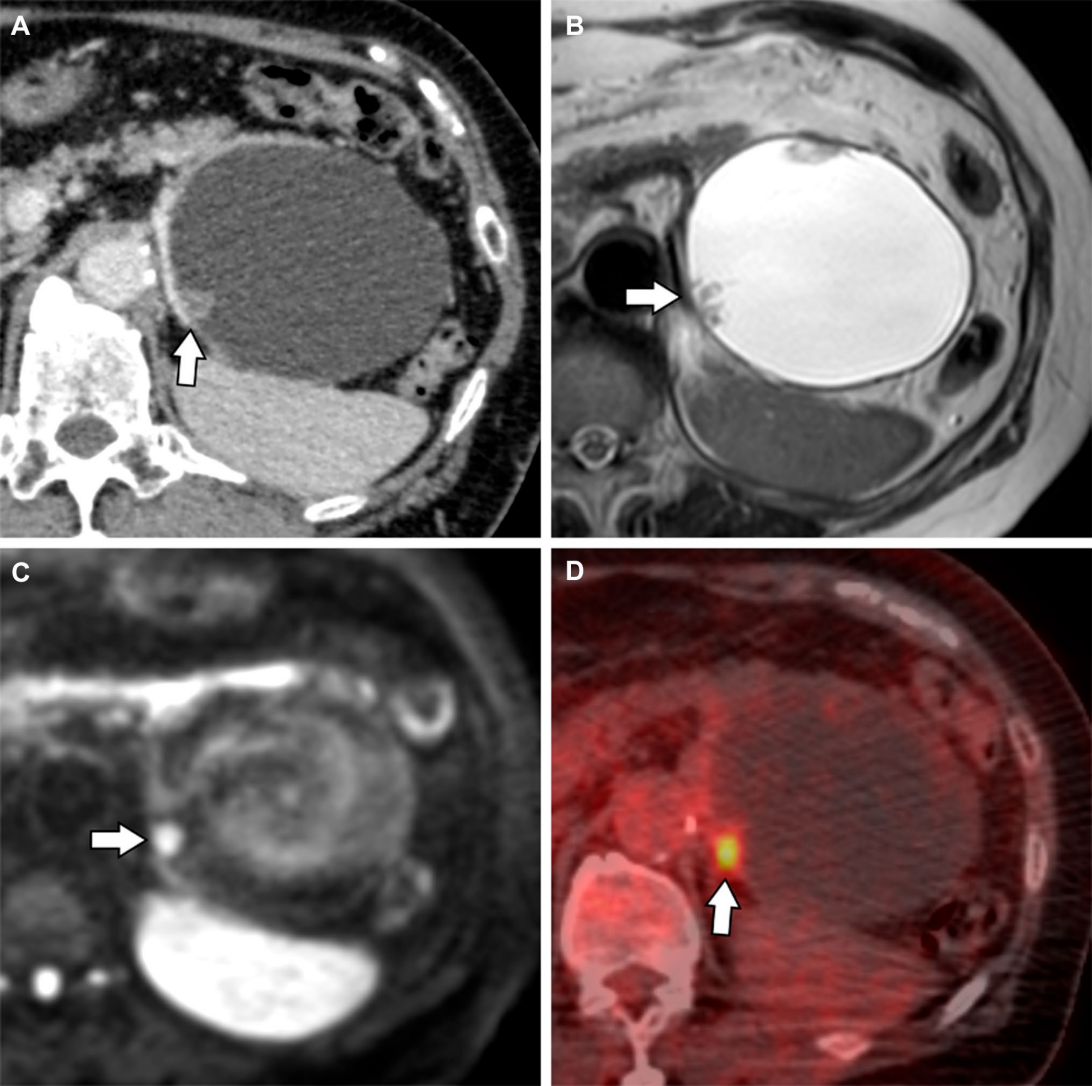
Case of a 66-year-old woman with a chief complaint of abdominal distension shows a mass in the tail of the pancreas (Case 11). A 91-mm-diameter cystic lesion is observed in the tail of the pancreas, and a contrast-enhanced 10-mm-diameter mural nodule is observed on contrast-enhanced computed tomography (a: arrow). On T2-weighted and diffusion-weighted images, mural nodule shows high signal intensity (b,c: arrow). ^18^F-fluorodeoxyglucose (FDG)-positron emission tomography/computed tomography shows accumulation in the mural nodule (d: arrow).
